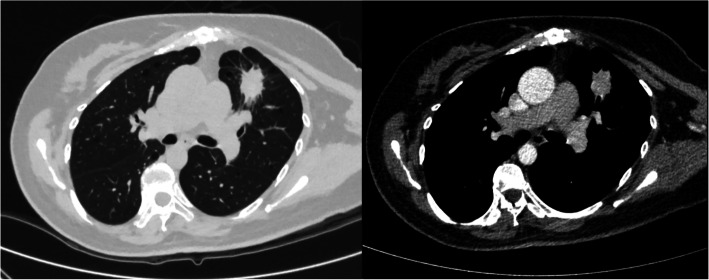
Chest CT at our hospital showed an irregular soft tissue mass in the anterior segment of the upper lobe of the left lung
